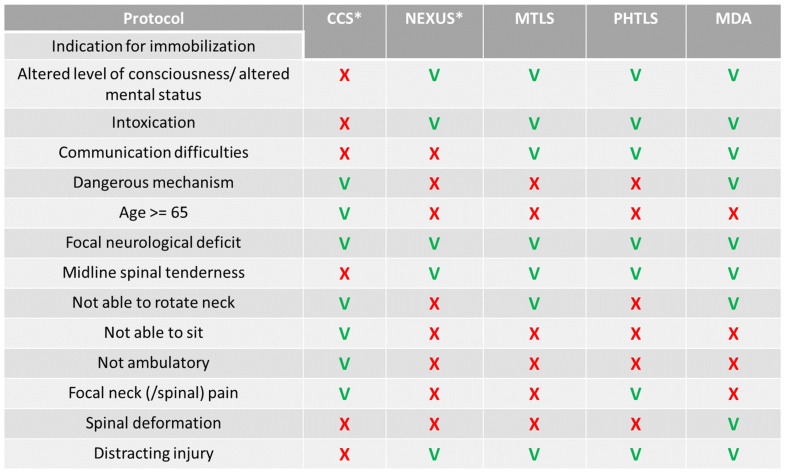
Comparison of cervical spine management protocols. CCS = Canadian cervical spine rule; NEXUS = national emergency X-radiography utilization study; MTLS = military trauma life-support protocols of the Israeli Defense Forces; PHTLS = prehospital life-support protocols; MDA = Magen David Adom (the Israeli civilian organization for prehospital care) protocols. (*) The CCS and the NEXUS criteria are meant to indicate spinal imaging in-hospital; the MTLS, PHTLS, and MDA protocols are meant to indicate spinal immobilization in the prehospital setting.
